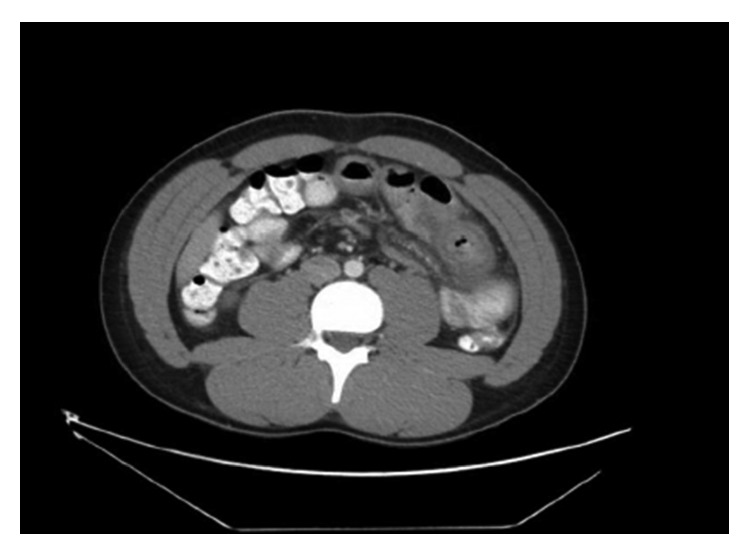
Abdominal computed tomography scan showing thickening of the small bowel wall.
